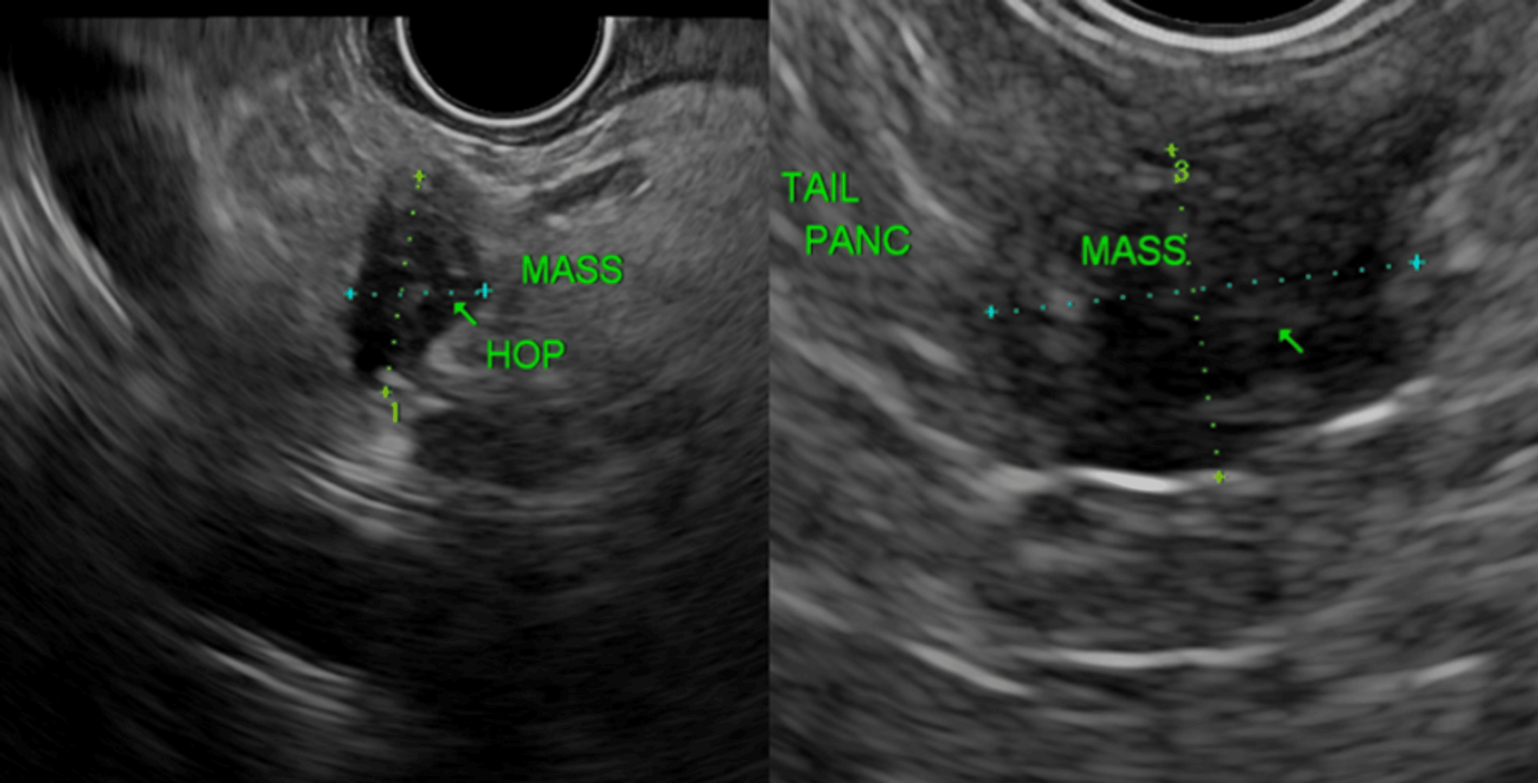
Endoscopic ultrasound (EUS) showing mass in head and tail of pancreas
